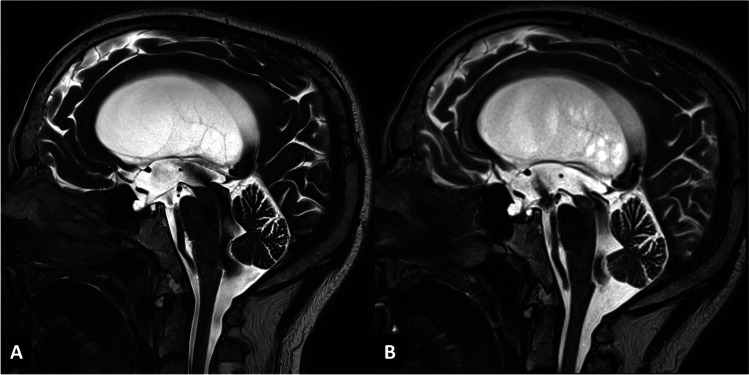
Preoperative and postoperative (4 months of follow-up) T2-weighted MRI in midsagittal view of a LOVA patient from our cohort. Some aforementioned peculiar characteristics are reported, especially a severe ventriculomegaly with an enlarged cisterna magna (A). The follow-up imaging shows an adequate CSF flow through the ventriculostomy in the third ventricle floor and a slightly less turbulent flow through the aqueduct, when compared to the preoperative MRI (B)
